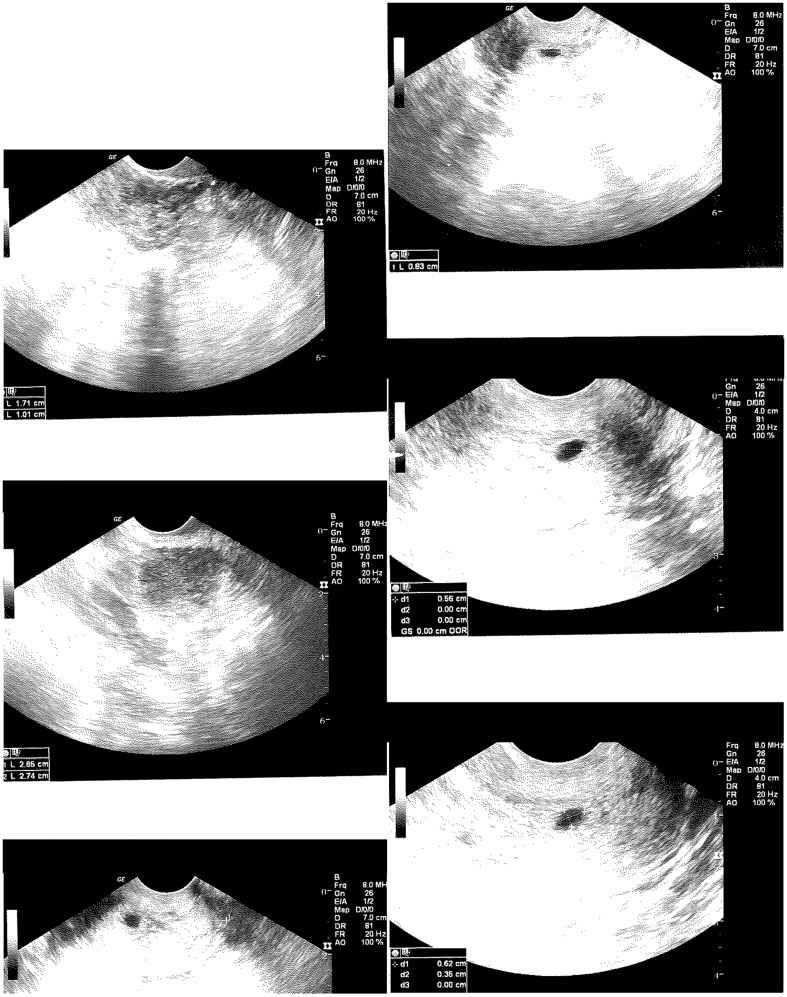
Transvaginal ultrasound at 5 weeks and 2 days of gestation. Gestational sac measuring 8.3 mm in the upper cervix. No yolk sac or fetal pole visualized. Beta-hCG 4607 mIU/mL.
